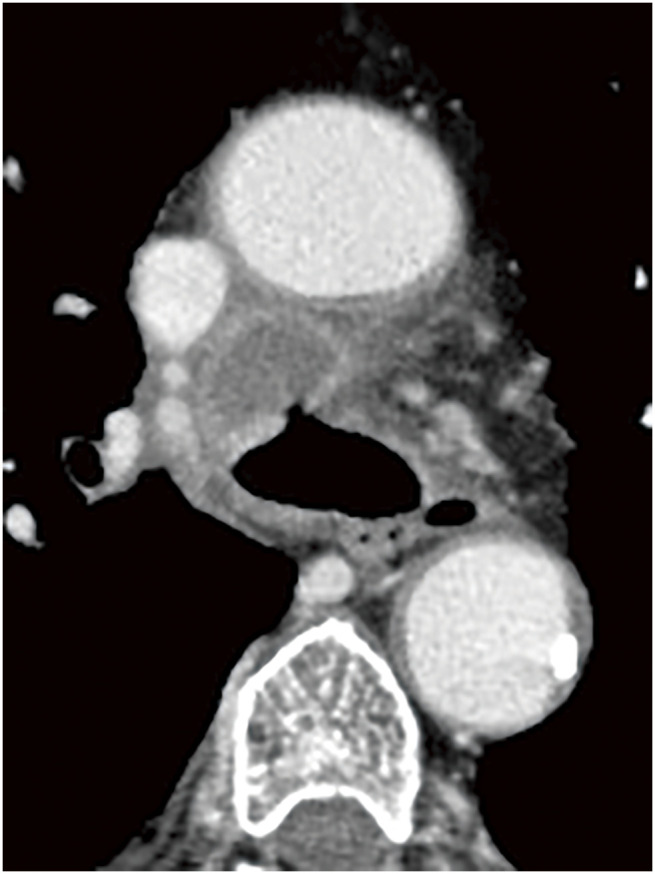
CT images taken at the time of fistula formation showed a low-attenuation area connected to the fistula in front of the carina, which was surrounded by ring-like structures that showed contrast enhancement. CT, computed tomography
